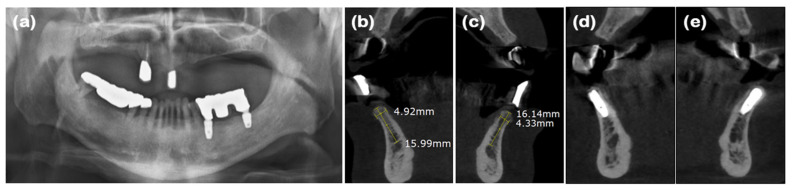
Radiographs of case 3. (a) The pre-operative panoramic view taken during the initial visit. (b,c) CBCT images displaying the augmented site after healing. The alveolar ridge width was measured at approximately 4.92 and 4.33 mm on the mandibular right and left lateral incisor areas, respectively, which is sufficient for the placement of a standard diameter implant. (d,e) The implants were placed in the mandibular right and left lateral incisor areas, respectively.
